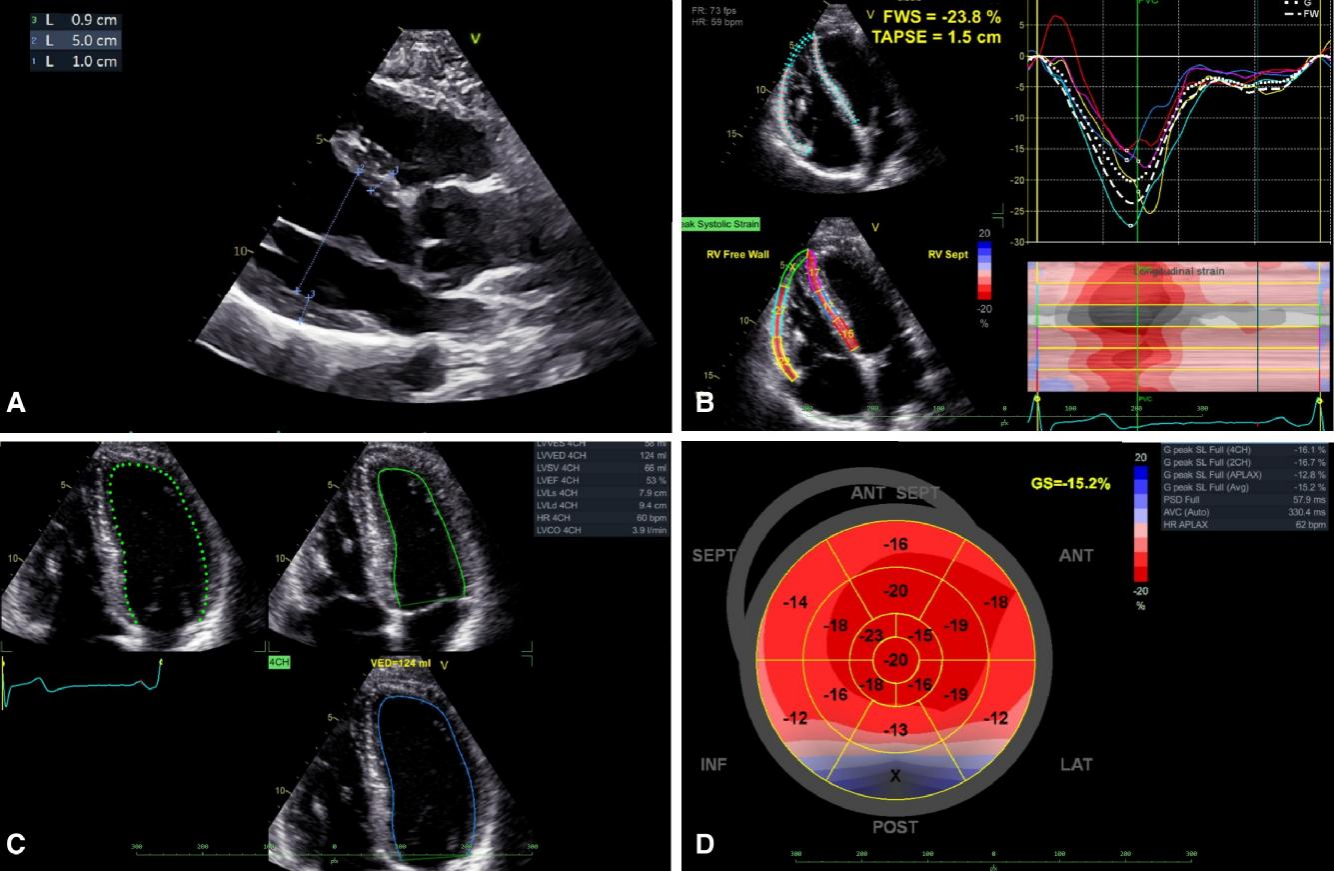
Echocardiographic examination: (*A*) long axis with normal left-sided heart compartments and aorta. (*B*) Normal non-dilated right ventricular function. (*C*) Normal left ventricular function. (*D*) No regional kinetics disturbance.
